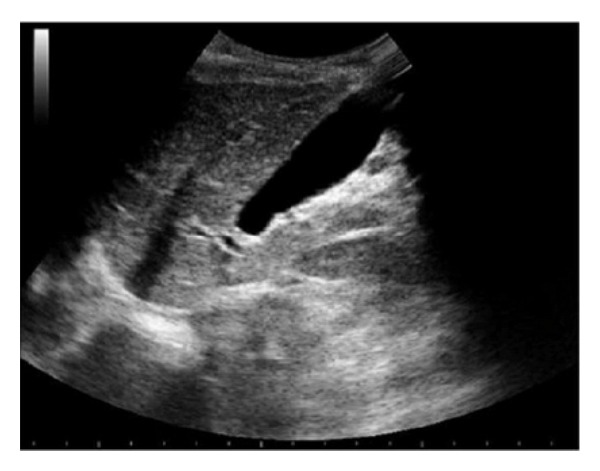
Hepatic ultrasound showing a distended gallbladder.
